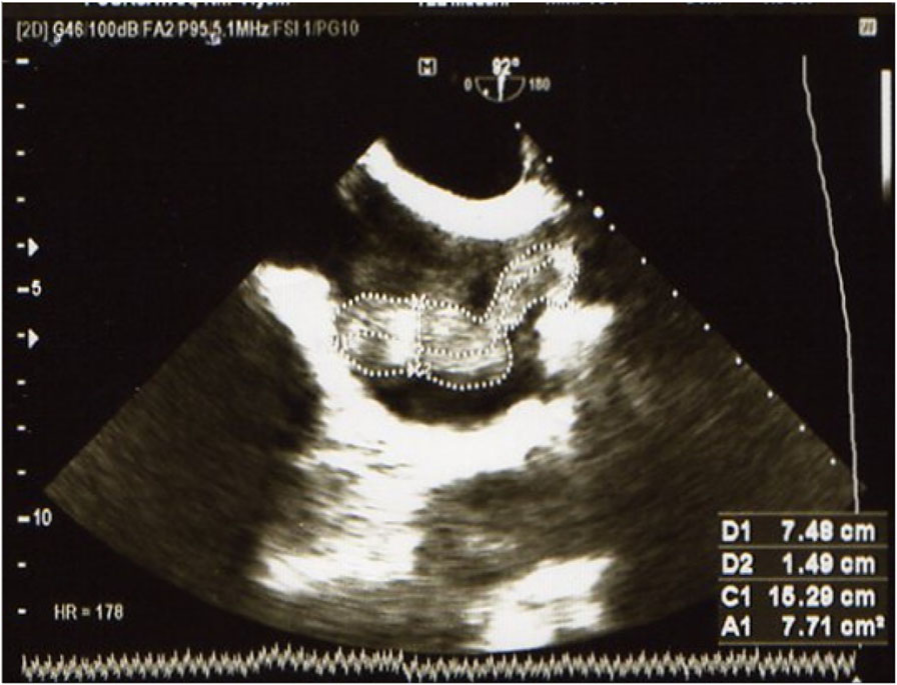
Bicaval view in transesophageal echocardiography showing a huge multilobulated mass in the right atrium and involvement of the upper part of the superior vena cava
